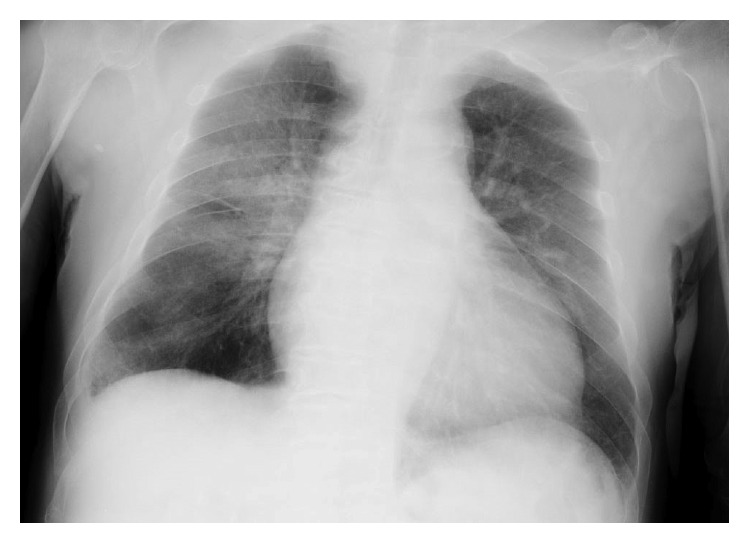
Chest X-ray findings on admission. Image shows alveolar opacity in both lungs, particularly in upper and middle lobes.
